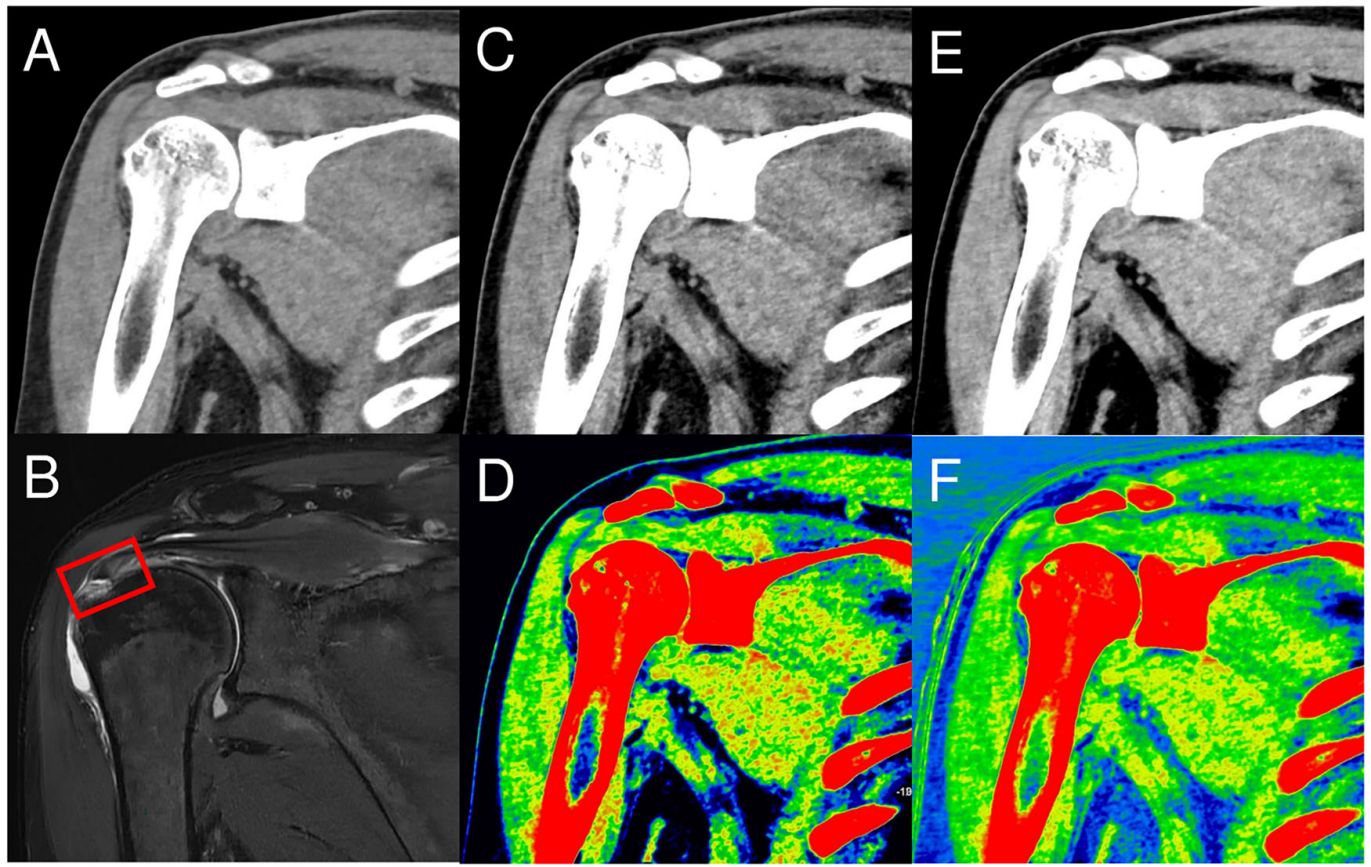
Oblique coronal plane images of a 49-year-old man with right supraspinatus tendon tear: (A) SCT image, (B) proton density weighted fat-saturated MRI image showing a tear in the supraspinatus tendon (red dotted rectangle), (C) DECT images with mono+ 50 keV, (D) color-coded DECT images with mono+ 50 keV, (E) DECT images with mono+ 90 keV, and (F) color-coded DECT images with mono+ 90 keV. SCT, standard computed tomography; DECT, dual-energy computed tomography; MRI, magnetic resonance imaging
